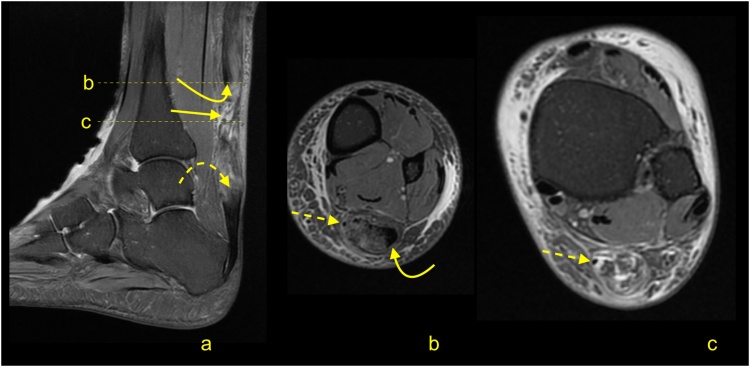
Achilles tendon rupture in midportion (straight arrow), 8 weeks after trauma. MRI (a–c) revealed fibrotic adhesions between the proximal stump (curved arrow) and distal stump (dashed arrow). The plantaris tendon is preserved (dashed arrow). A, b and c- PD- weighted with fat suppression.
